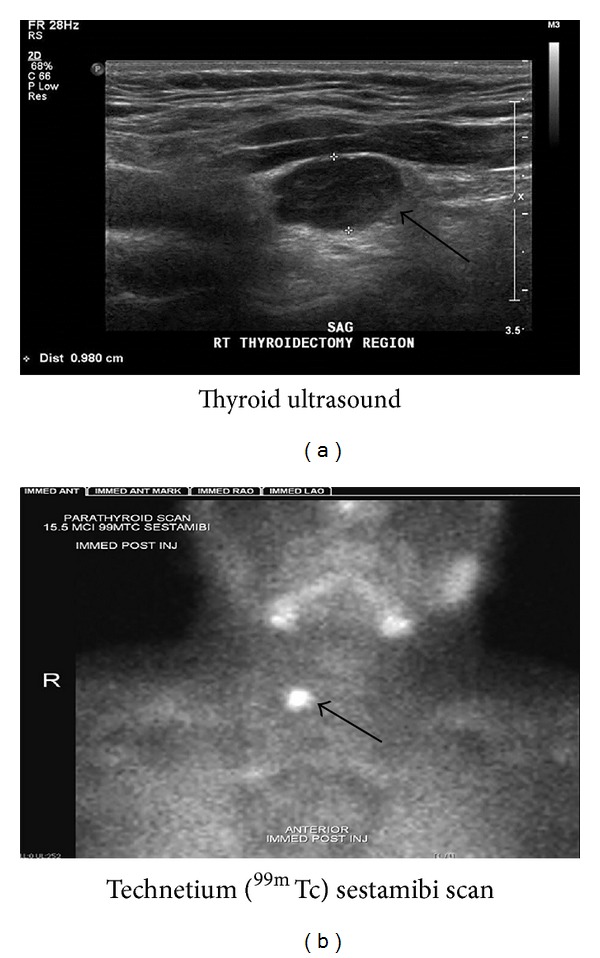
Ultrasound (a) and technetium (^99m^Tc) sestamibi scan (b) of nonpalpable 1 cm nodule in right neck prior to removal of parathyroid adenoma.
